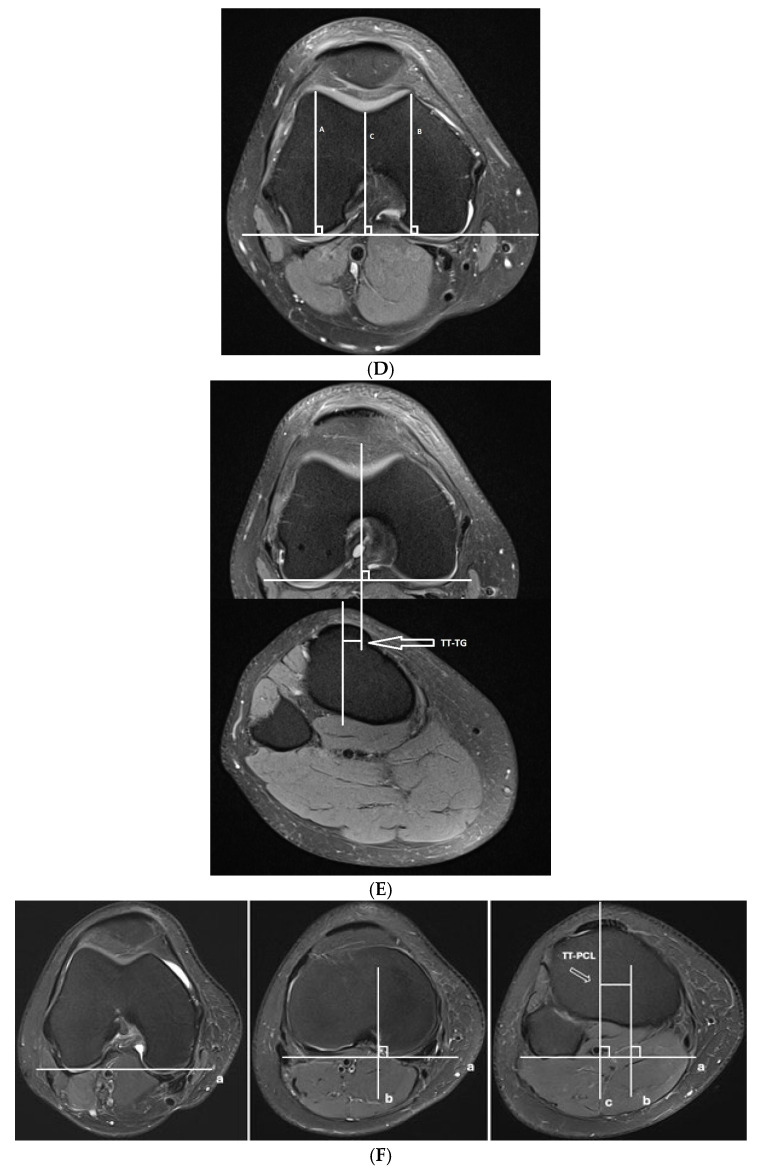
MRI measurements for the assessment of patellar dislocation. (A) Lateral trochlear inclination (LTI), measured between the bony contours of the lateral trochlear facet on the most proximal axial slice containing trochlear cartilage and a posterior condylar tangential line at the same level. (B) Lateral trochlear inclination modified (LTI m), measured between the bony contours of the lateral trochlear facet at the level of the trochlear groove and a posterior condylar tangential line at the same level. (C) Sulcus angle (SA), measured between the medial and lateral facets using bony landmarks for measurement purposes. (D) Trochlear depth (TD), assessed by measuring the maximal anteroposterior distance of the medial femoral condyle (distance A), the maximal anteroposterior distance of the lateral femoral condyle (distance B), and the minimal anteroposterior distance between the deepest point of the trochlear groove and the line paralleling the posterior outlines of the femoral condyles (distance C), all measured on the axial scan about 3 cm above the joint line. TD was calculated according to the following formula: ([A + B]/2) − C. (E) Tibial tubercle–trochlear groove distance (TT-TG): the maximum distance taken from the lines drawn perpendicular to the deepest area of the trochlear groove and the center of the patellar tendon insertion on the tibial tuberosity on axial images. (F) Tibial tuberosity–posterior cruciate ligament distance (TT-PCL): the maximum distance taken from the lines drawn perpendicular to the medial border of the posterior cruciate ligament at its tibial insertion (b) and the center of the patellar tendon insertion on the tibial tuberosity on axial images (c), parallel to the posterior condylar tangential line (a).
